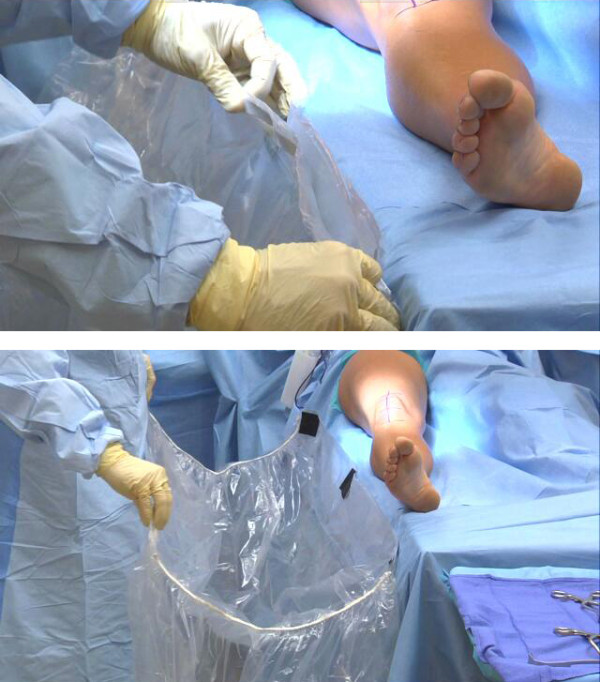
Expandable Collapsible Drape. The drape lies flat to the table providing unencumbered access to the surgical site, it simply manually expands with release of the fastener tabs to except the x-ray tube. The sterile pouch lies adjacent to the patient. The pouch is radiolucent allowing improved fluoroscopy targeting. The pouch also protects the x-ray tube from biohazard fluids.
